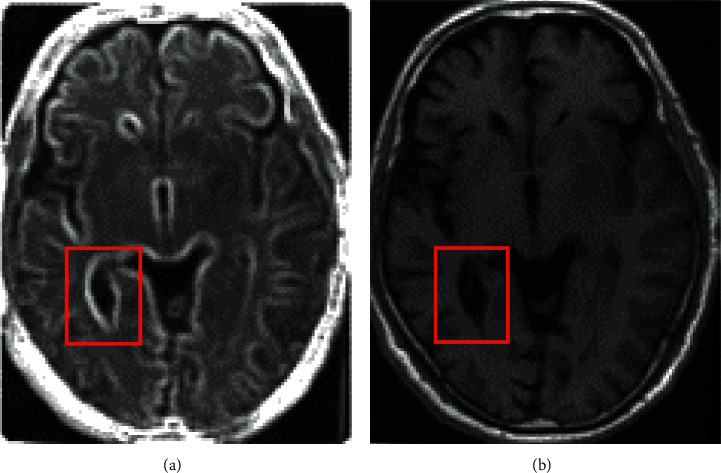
Comparison of MRI images before and after being processed by the GMM-based LRMD algorithm. (a) The image before denoising, and (b) the image after denoising. The red box indicates intracranial hemorrhage.
